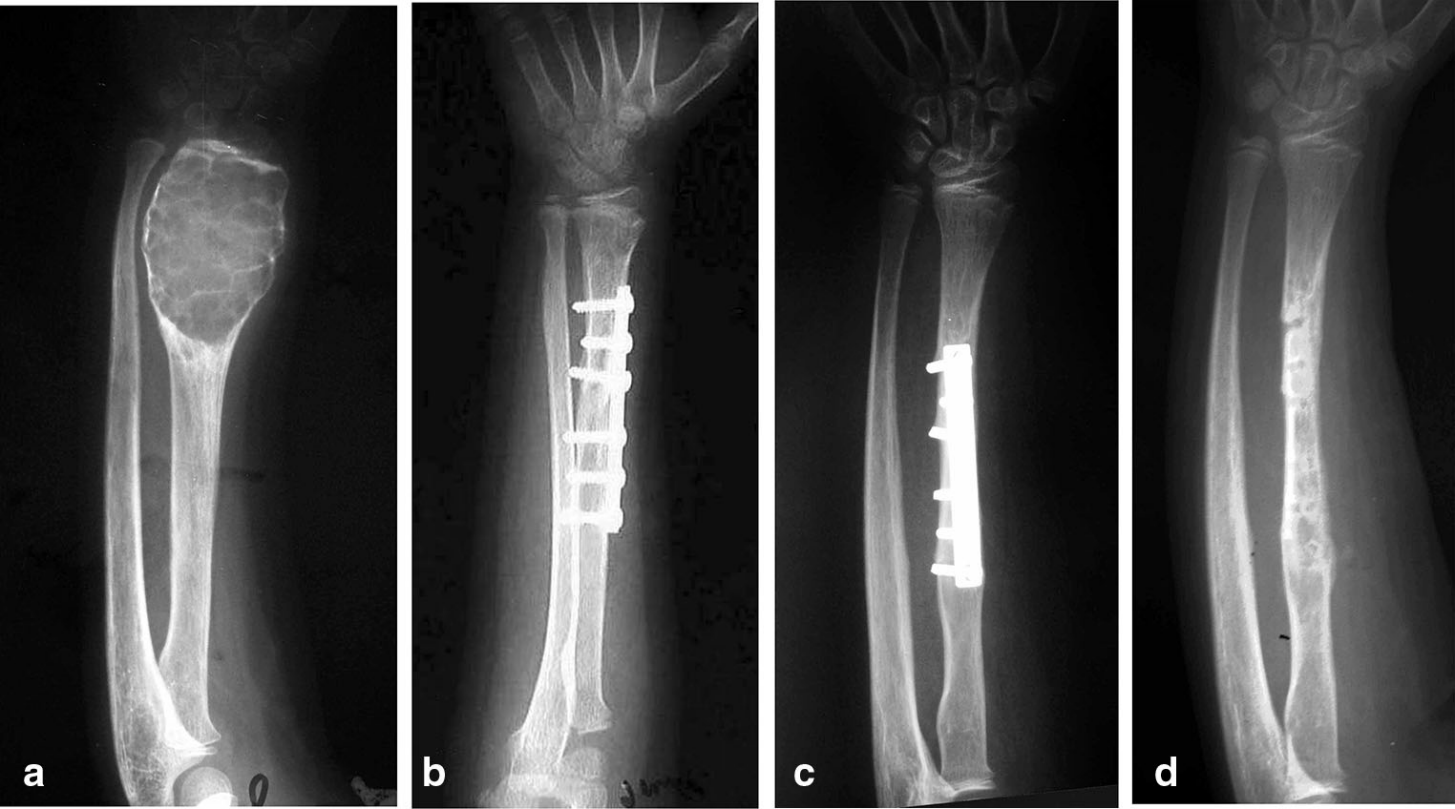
a Anteroposterior radiograph of a 6-year-old girl with ABC destructing the distal one-third of left radius (Case 1). b Proximal fibular graft was harvested from her mother, shaped and stabilized with plate and screws. c Follow-up radiograph showing continued growth of the graft and maintained open physis. d 5 years after surgery with continuing growth and no deformity
